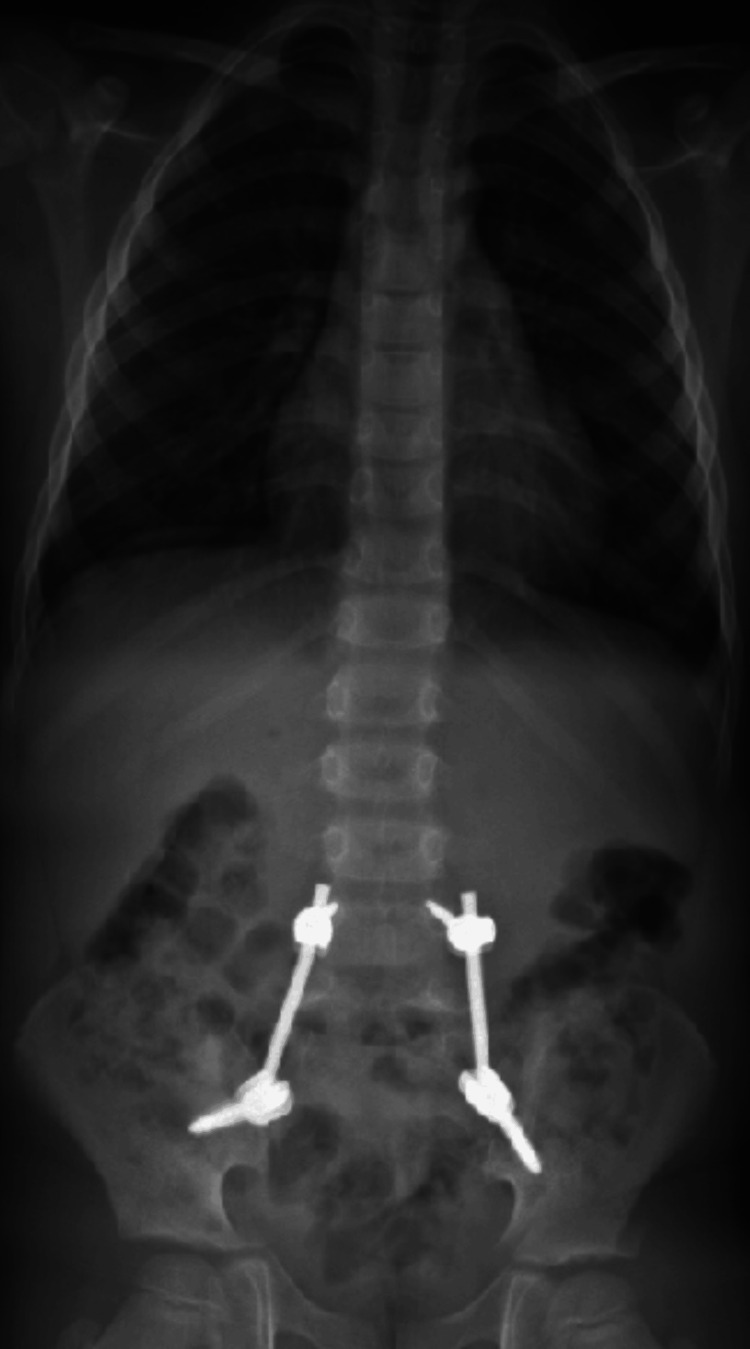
Radiograph at 12 months postoperatively
